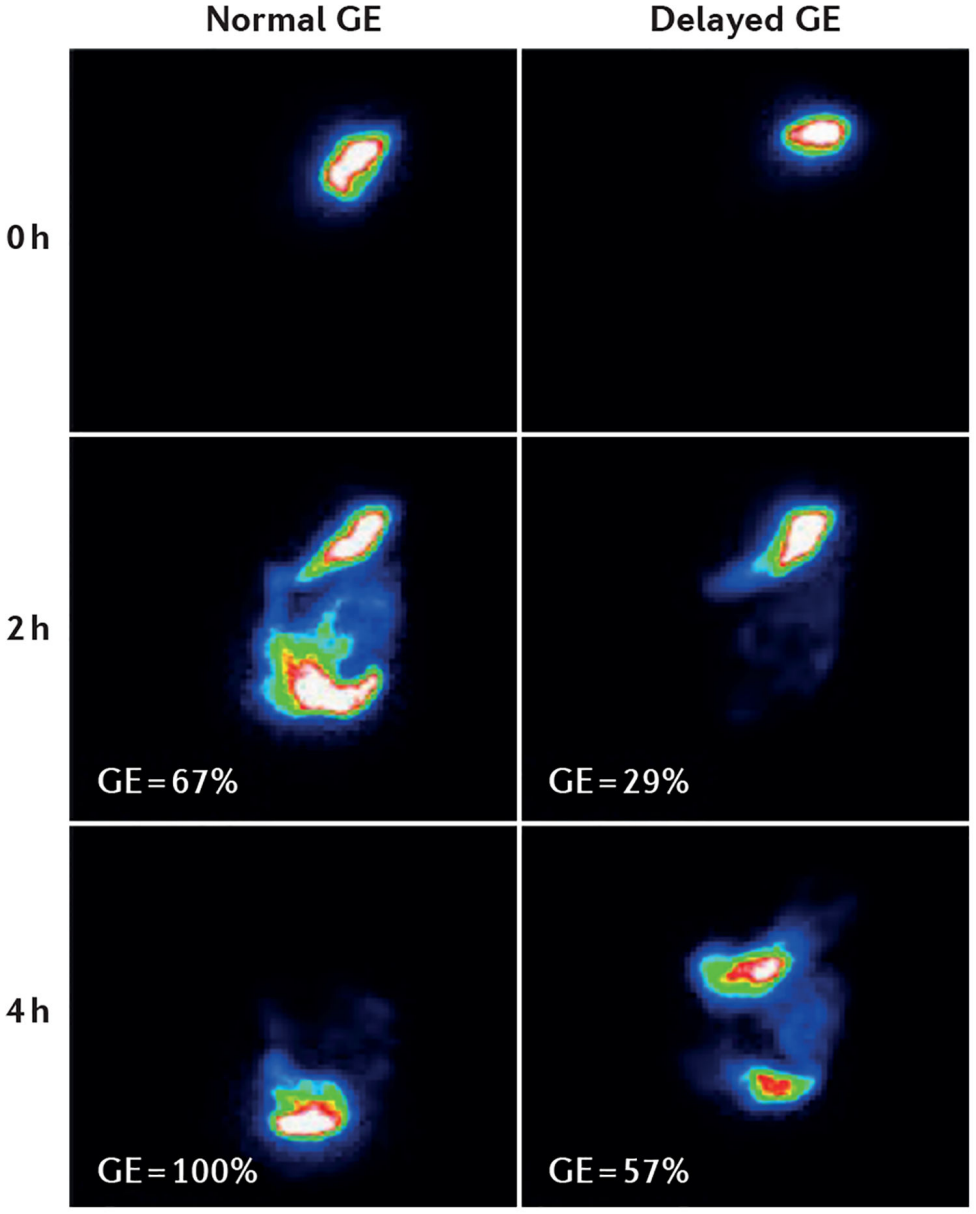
Representative examples of gastric emptying as assessed using scintigraphy. Standardized scintigraphic study of gastric emptying of solids with consumption of a 320 kcal radiolabelled meal (scrambled eggs labelled with ^99m^Tc; Mayo Clinic protocol^30^) and imaging over 4 h. In the individual with normal gastric emptying (GE) (left panel), large amounts of the meal are emptied from the stomach at 2 h, and GE is completed at4 h. In the individual with delayed GE (right panel), gastric retention of the test meal at 2 h and particularly at 4 h is increased (normative values were determined from 319 healthy volunteers; clinically relevant delayed GE is defined as a percentage retention >75% at 2 h and >25% at 4 h)^30^.
